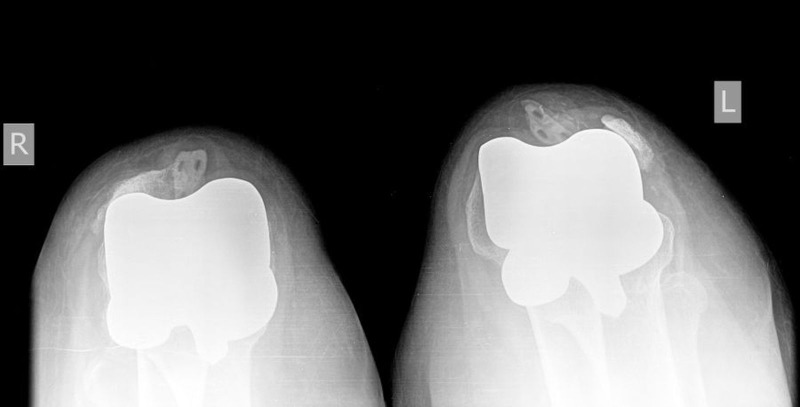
X-ray with skyline view of both knees showing component loosening, along with dislocation of the patella
